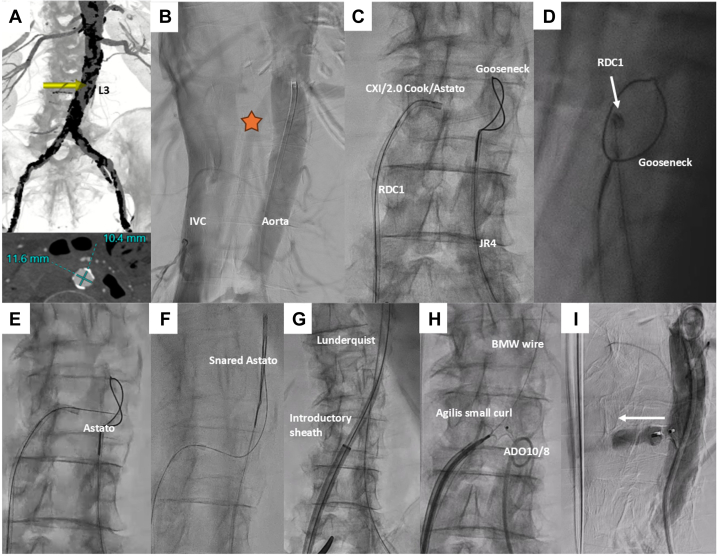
Transcaval TAVR (A) Preoperative computed tomography for evaluating feasibility and locating the traversal site. (B) Simultaneous aortogram and venogram to confirm the target puncture site. (C) Astato guidewire in the IVC and gooseneck snare in the aorta. (D) Orthogonal project to confirm the traversal site. (E) Astato wire traversal from the IVC to the aorta. (F) Astato wire snared in the aorta. (G) Delivery of TAVR sheath through the cavo-aortic access. (H) Closure of the cavo-aortic tract. (I) Aortogram to confirm satisfactory sealing of the cavo-aortic tract. ADO10/8 = amplatzer duct occluder 10/8; BMW = balance middleweight; IVC = inferior vena cava; JR4 = Judkins right 4; RDC1 = renal double-curve 1; other abbreviations as in Figure 1.
